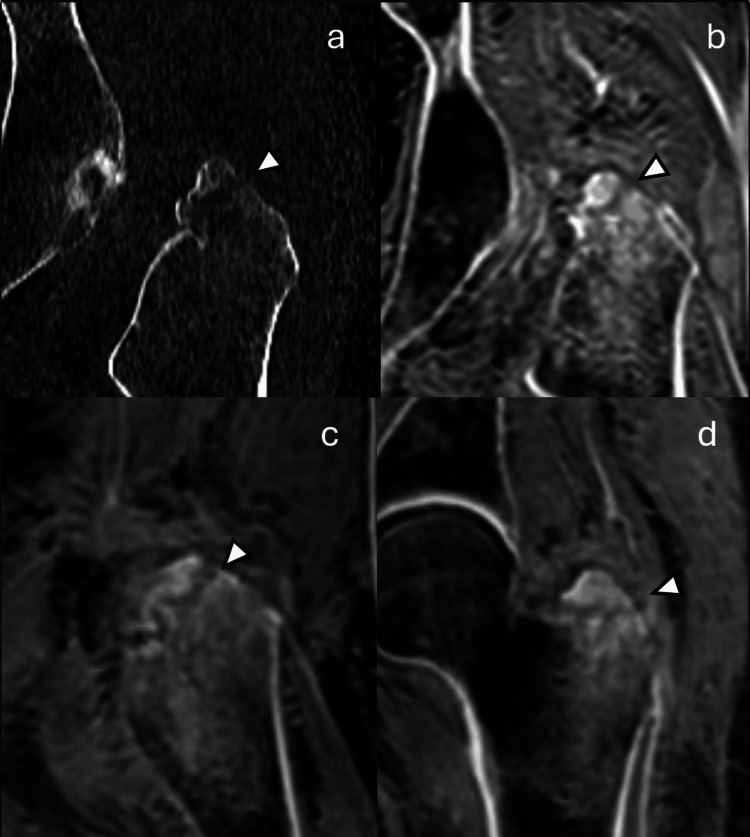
Representative imaging example 2. A patient with a left greater trochanter fracture (arrowheads) seen on (a) CT and (b) pseudo-CT. As with CT, pseudo-CT also allows multiplanar reformatting for more comprehensive fracture visualization and assessment (c, d).
